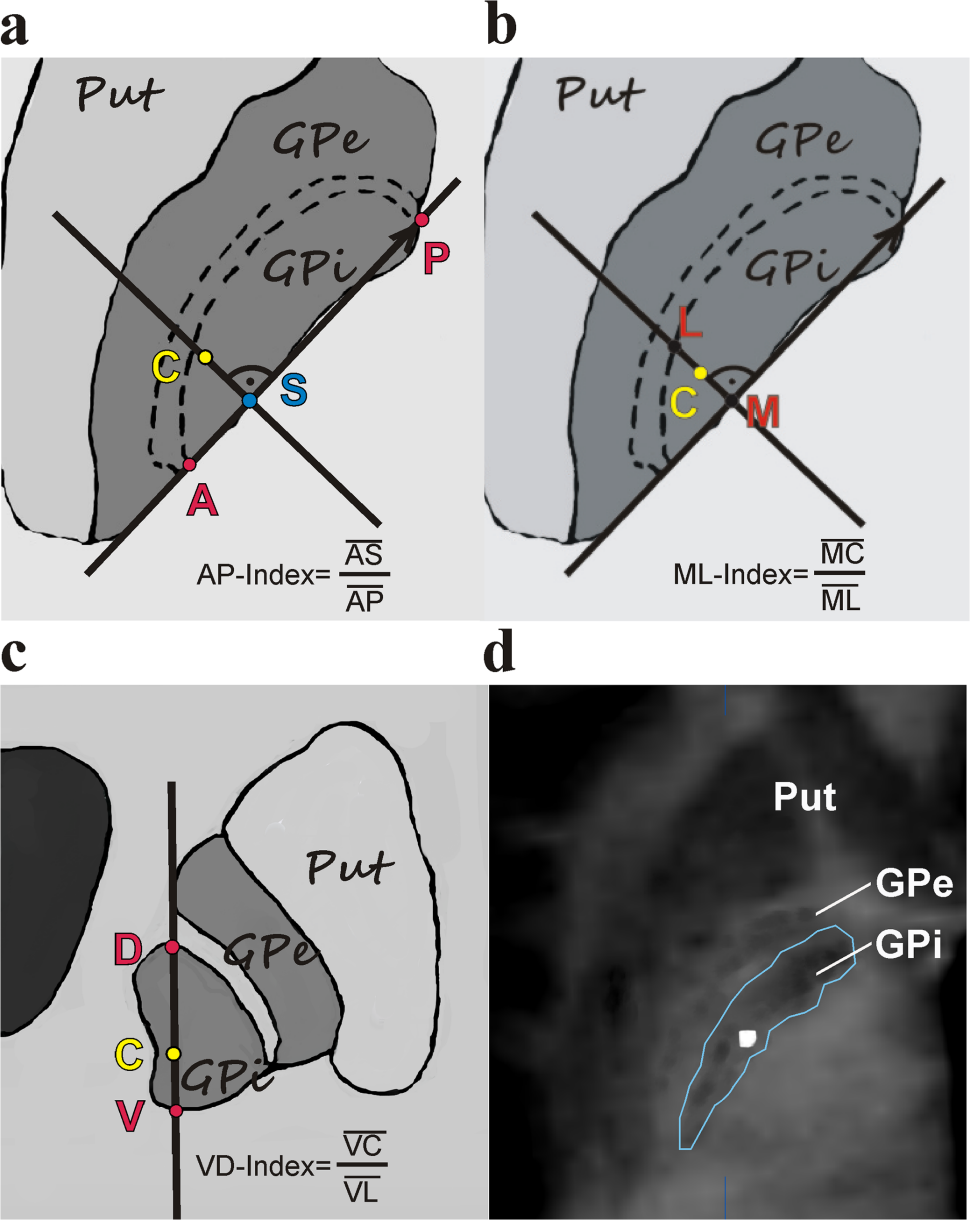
Determination of the AP-, ML- and VD-Index of the active contact C. A: A line between the most anterior (A) and posterior (P) points of the GPi constitutes the AP-line. Another line through the active contact C and perpendicular to AP intersects the AP-line in S. The AP-Index can be calculated as AP-Index = AS/AP. B: The medial (M) and lateral (L) point of the GPi are located on a line perpendicular to the AP-line crossing the active contact C. The ML-Index can be calculated as ML-Index = MC/ML. C: The ventral (V) and dorsal (D) point of the GPi are located on a line perpendicular to the ML-line crossing the active contact C. The VD-Index can be calculated as VD-Index = VC/VD. D: An overlay of the postoperative CT-scan at the level of the active contact and the MDEFT MRI sequence shows the border of the GPi after segmentation reveals the position of the active contact in relation to the anatomical borders of the GPi.
